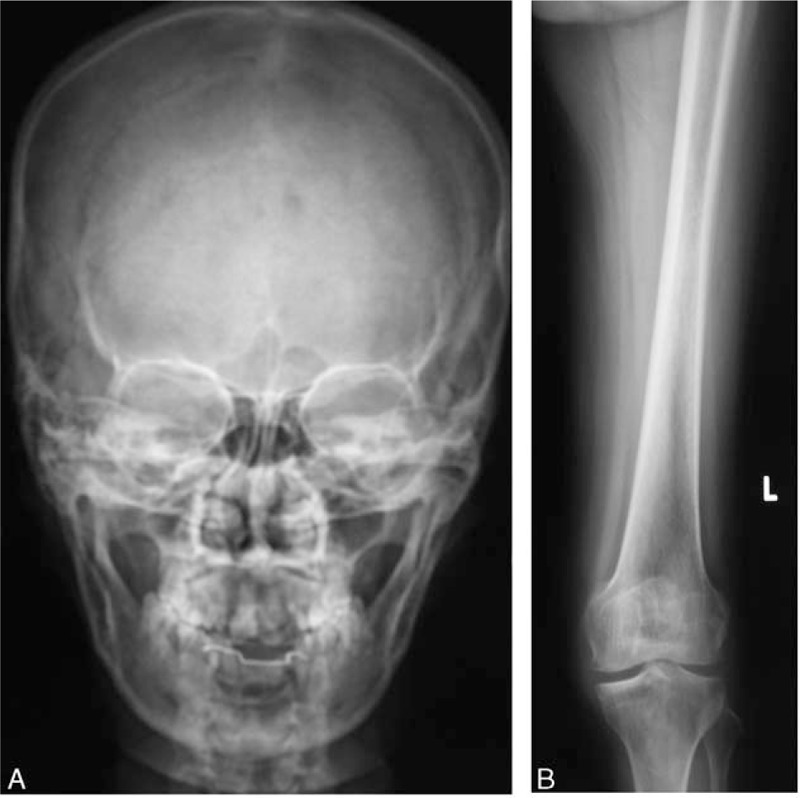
X-ray of skull (A) and left femur (B); X-ray of skull and left femur showed no osteolytic lesions.
